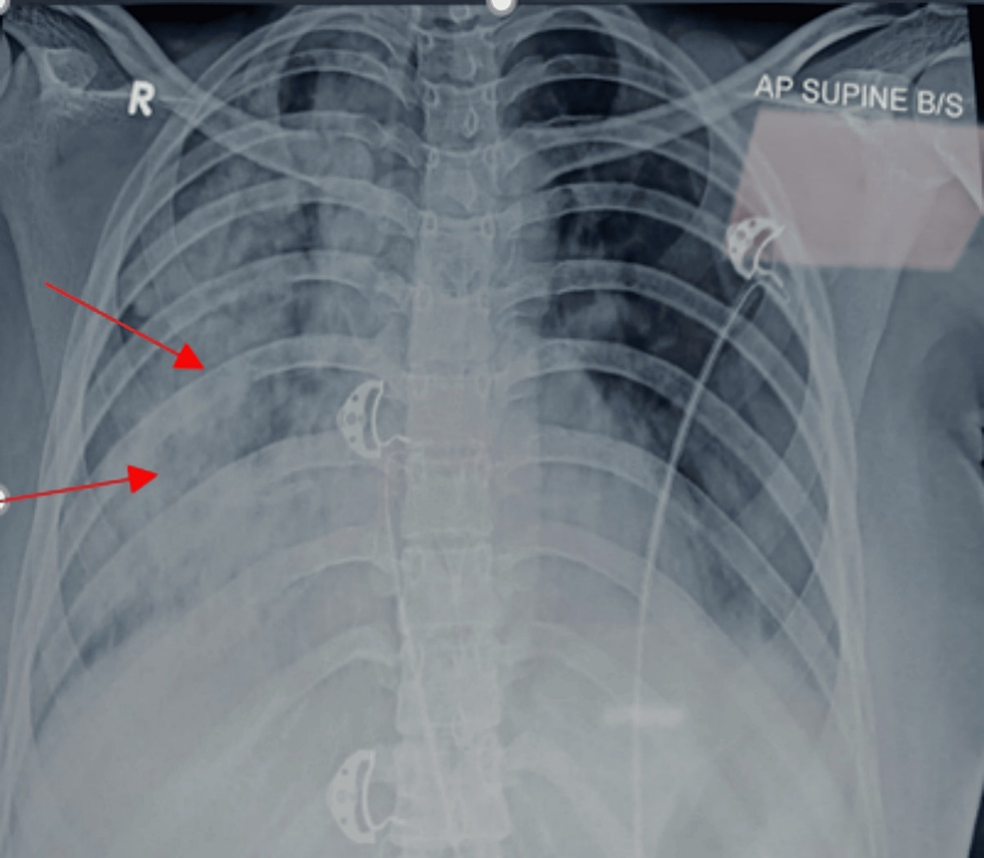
Chest X-ray showing unilateral lung infiltrates (red arrows)
